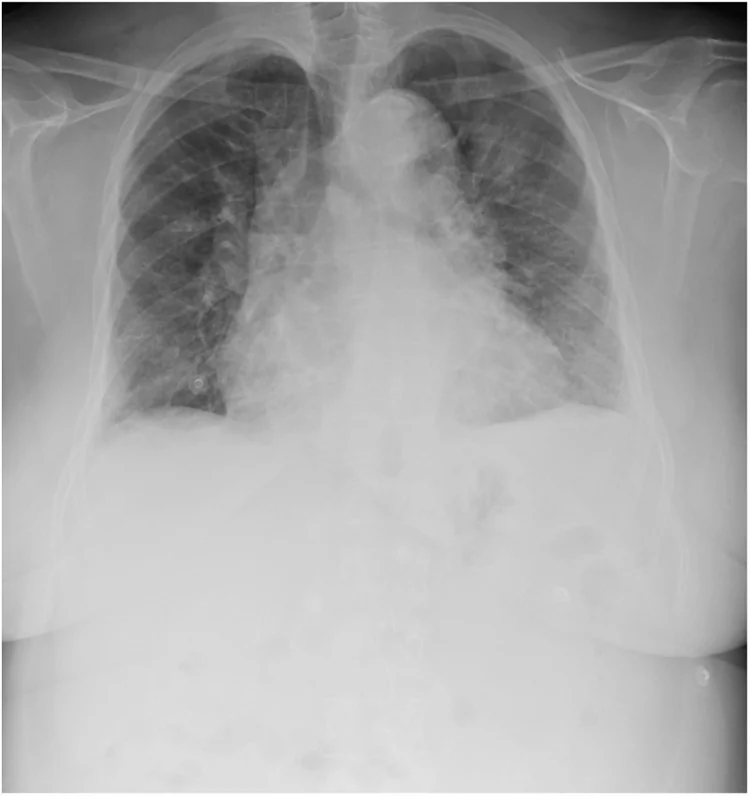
X-ray of diseased chest. Cite mimic cxr V2.1.0.
